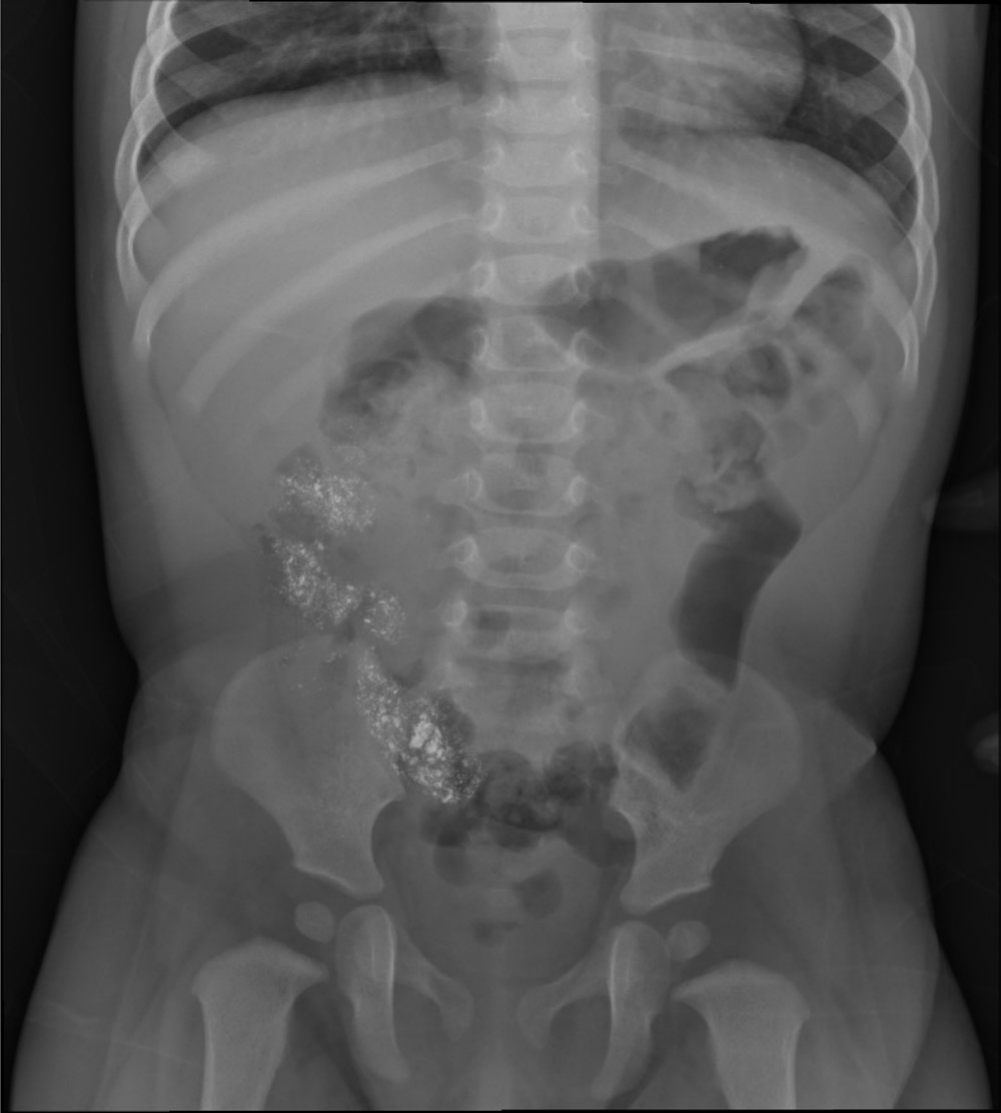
Abdominal radiograph approximately 7 hours after ingestion demonstrating hyperdense material in the ascending colon.
